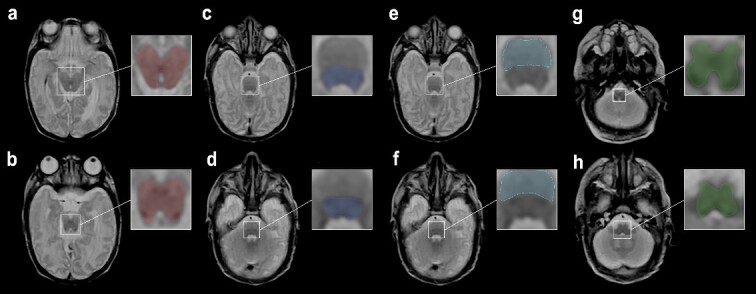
Female neonate with IVH grade III, born at 24 + 0 wk GA. MRI performed 93 days postpartum. ROIs were drawn on an MDME-based T2 contrast on a transversal plane. ROIs of the midbrain at the level of the a) superior colliculi b) inferior colliculi. c) Pontine tegmentum and e) basis pontis at the level of the superior olive. d) Pontine tegmentum and f) basis pontis at the level of the vestibular nuclei. Medulla oblongata at the level of the g) medial lemniscus (decussation) and h) inferior olivary nucleus.
